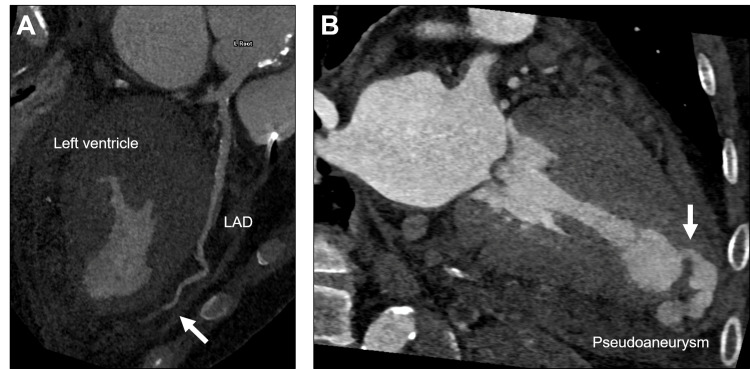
The preoperative cardiac computed tomography image Panel A shows a curved planar reconstruction image showing severe stenosis in the distal part of the left anterior descending artery indicated by the arrow. Panel B shows a long-axis view of the left ventricle showing pseudoaneurysms with a bead-like appearance. The arrow indicates the orifice of the pseudoaneurysm.
